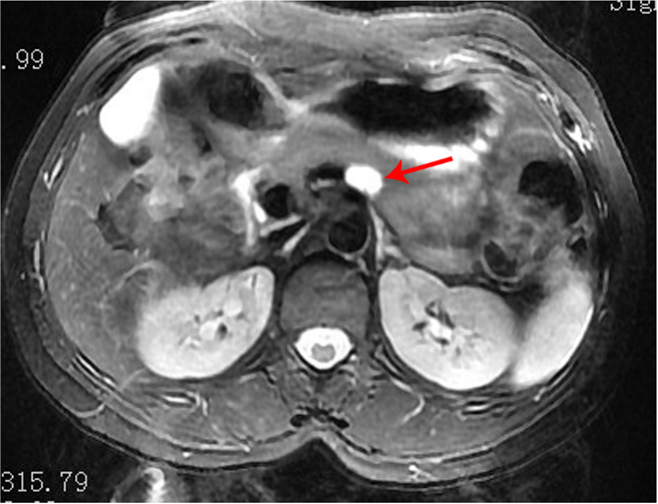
Magnetic resonance imaging. There is a 10 × 14-mm cystic neoplasm (arrow) in the proximal body of the pancreas with a clear-margin multilocular cavity and enhanced internal septum.
